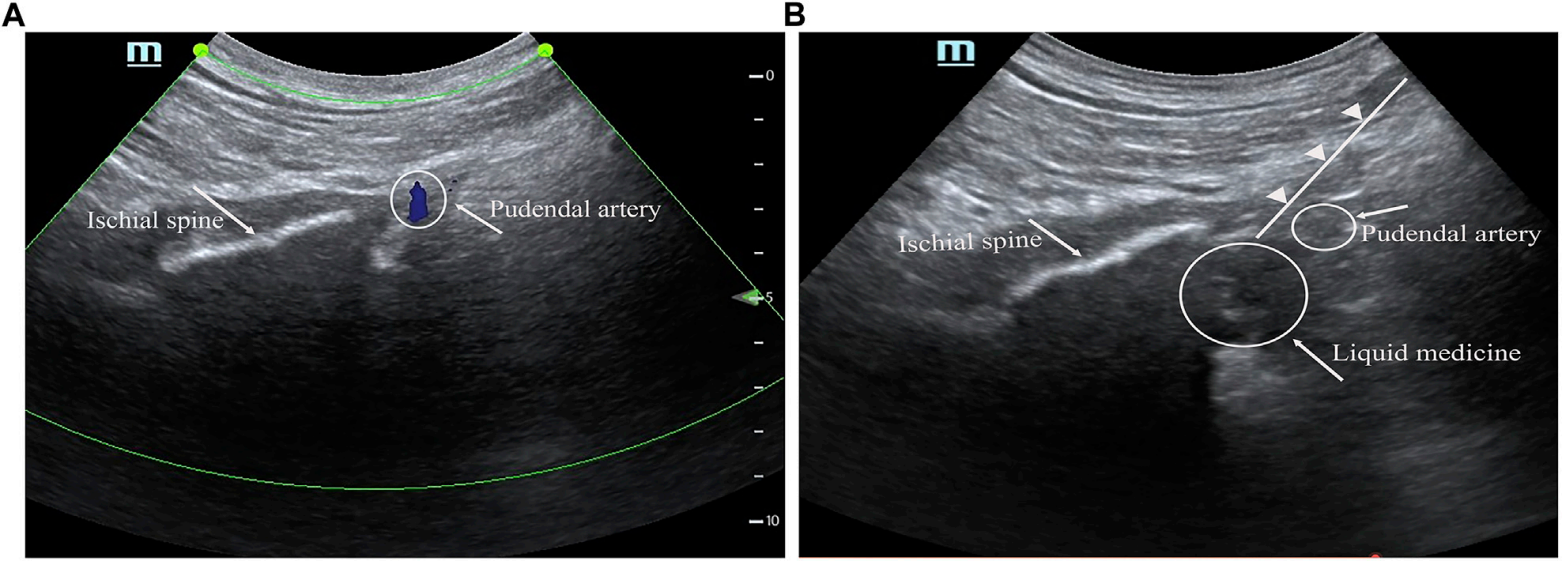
Ultrasound guided pudendal nerve block. (A): Positioning image before injection (B): image after injection. The white line represents the ischial spine, the smaller circle is the pudendal artery, the larger circle is the medicine, and the white triangle shows the needle’s trajectory.
